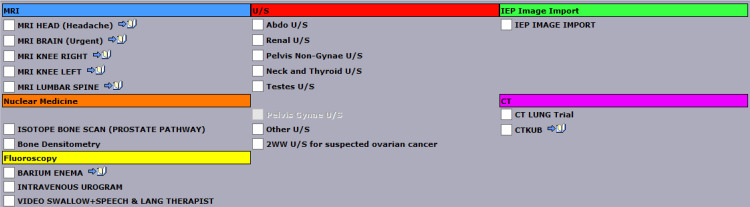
All the scans (except for plain film X-rays) available for direct access scan in a General Practice in Nottingham as of 6th of November 2023.
